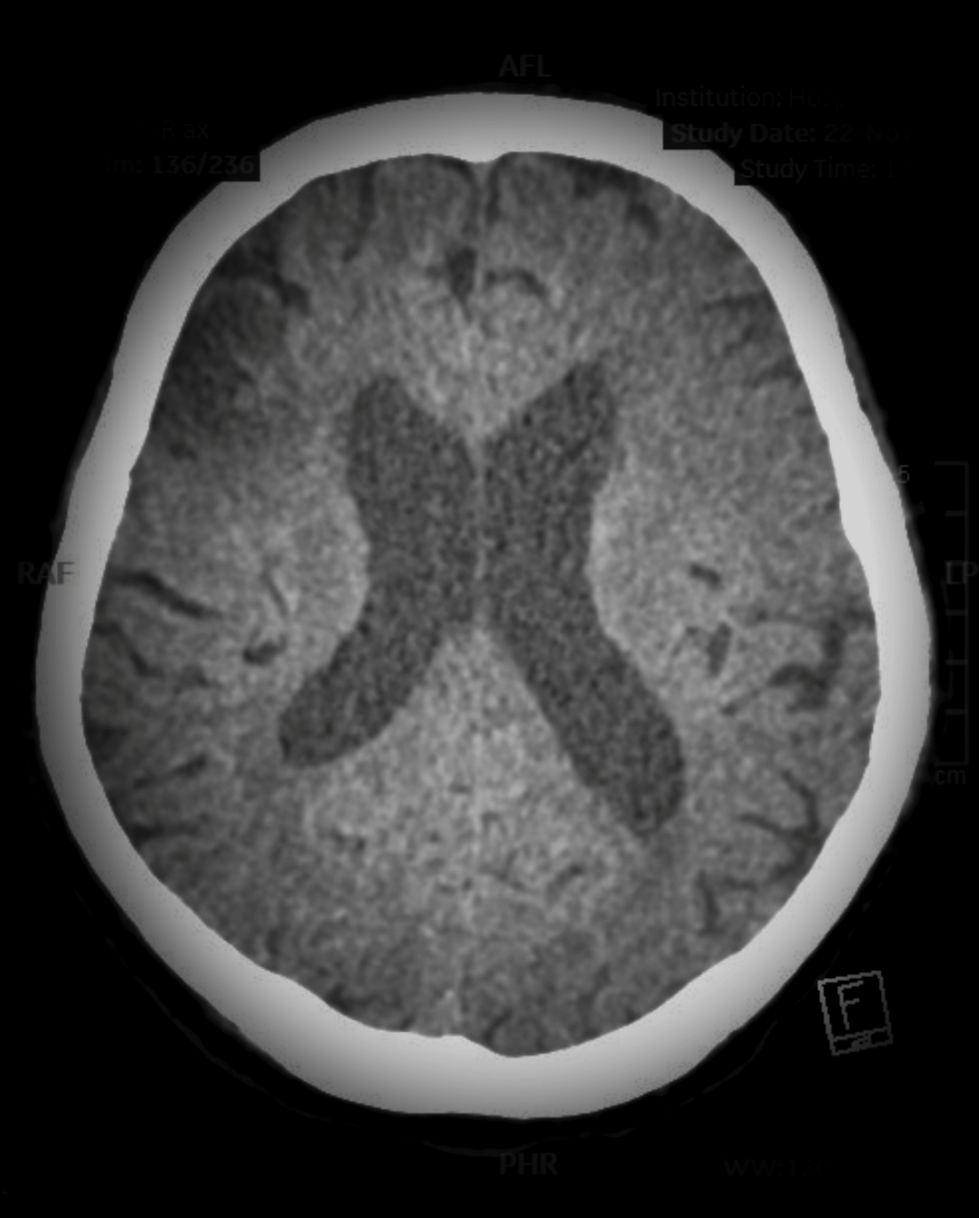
CT of the brain CT of the brain revealed acute infarct in the right middle cerebral artery territory, along with encephalomalacia in the right frontal and left temporo-occipital lobes. CT, computed tomography
